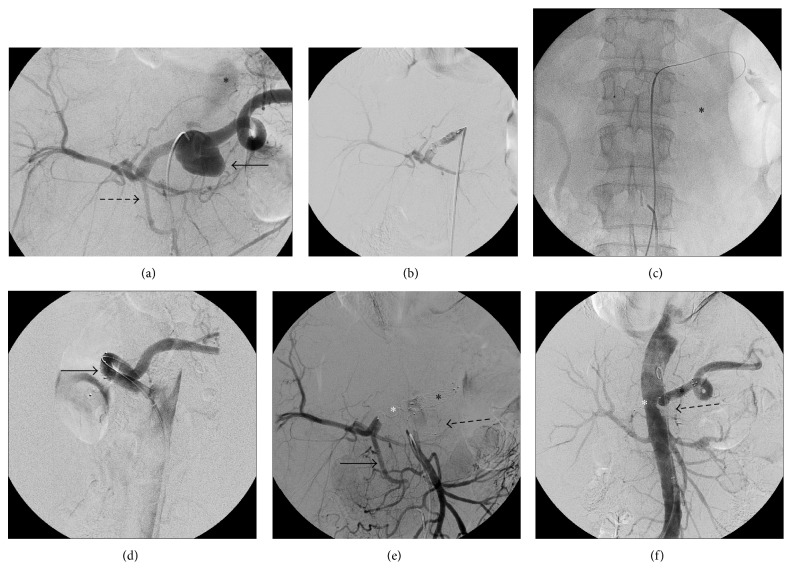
Diagnostic and procedural angiography. (a) Contrast injection from the celiac trunk confirming CT scan report: aneurysm of the celiac trunk (black arrow), cranial dissection, and consequent pseudoaneurysm (asterix) presenting with delayed enhancement and ectatic pancreaticoduodenal arcade (dashed black arrow); (b) release of a 12 mm vascular plug in the proximal third of the common hepatic artery; ((c)-(d)) positioning of a microcatheter (asterix) inside the aneurysm sac and of a guidewire in the splenic artery for the following release of a 12 × 80 mm covered stentgraft (black arrow); (e) contrast injection from the superior mesenteric artery showing the pancreaticoduodenal arcade (solid black arrow) supporting liver vascularization after occlusion of the hepatic artery with a vascular plug (white asterix), stent in the splenic artery (black asterix), and microcatheter inside the aneurysm sac (dashed black arrow); (f) aortography showing the complete exclusion of the aneurysm sac fulfilled with Onyx (dashed black arrow), patency of the covered stent positioned in the splenic artery (black asterix), and occlusion of the hepatic artery with a vascular plug (white asterix).
